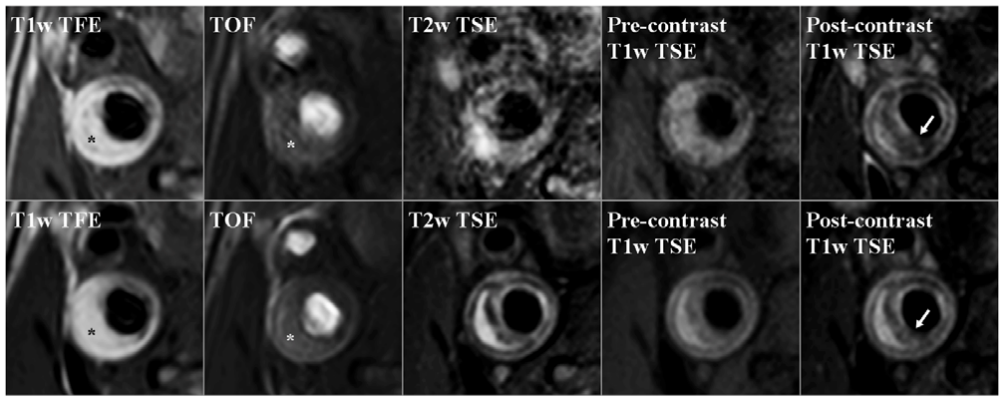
Multisequence MR images of a plaque in the internal carotid artery obtained at baseline (upper row) and after one year (bottom row). The TOF and T2w TSE images at baseline are slightly blurred due to patient motion. There is no obvious change of plaque morphology. There is a plaque with a LRNC and IPH (asterisks) at baseline and at 1-year follow-up. There is a disruption of the FC at both time points (arrows).
